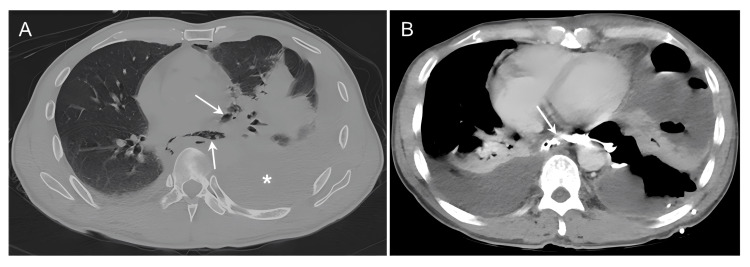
CT esophagography shows pneumomediastinum and perforation location. A, CT shows left hydropneumothorax (asterisk) and pneumomediastinum (arrow). B, CT scan with oral contrast agent (CA) revealed a fistula between the esophagus and the mediastinal paraoesophageal abscess (arrow).
